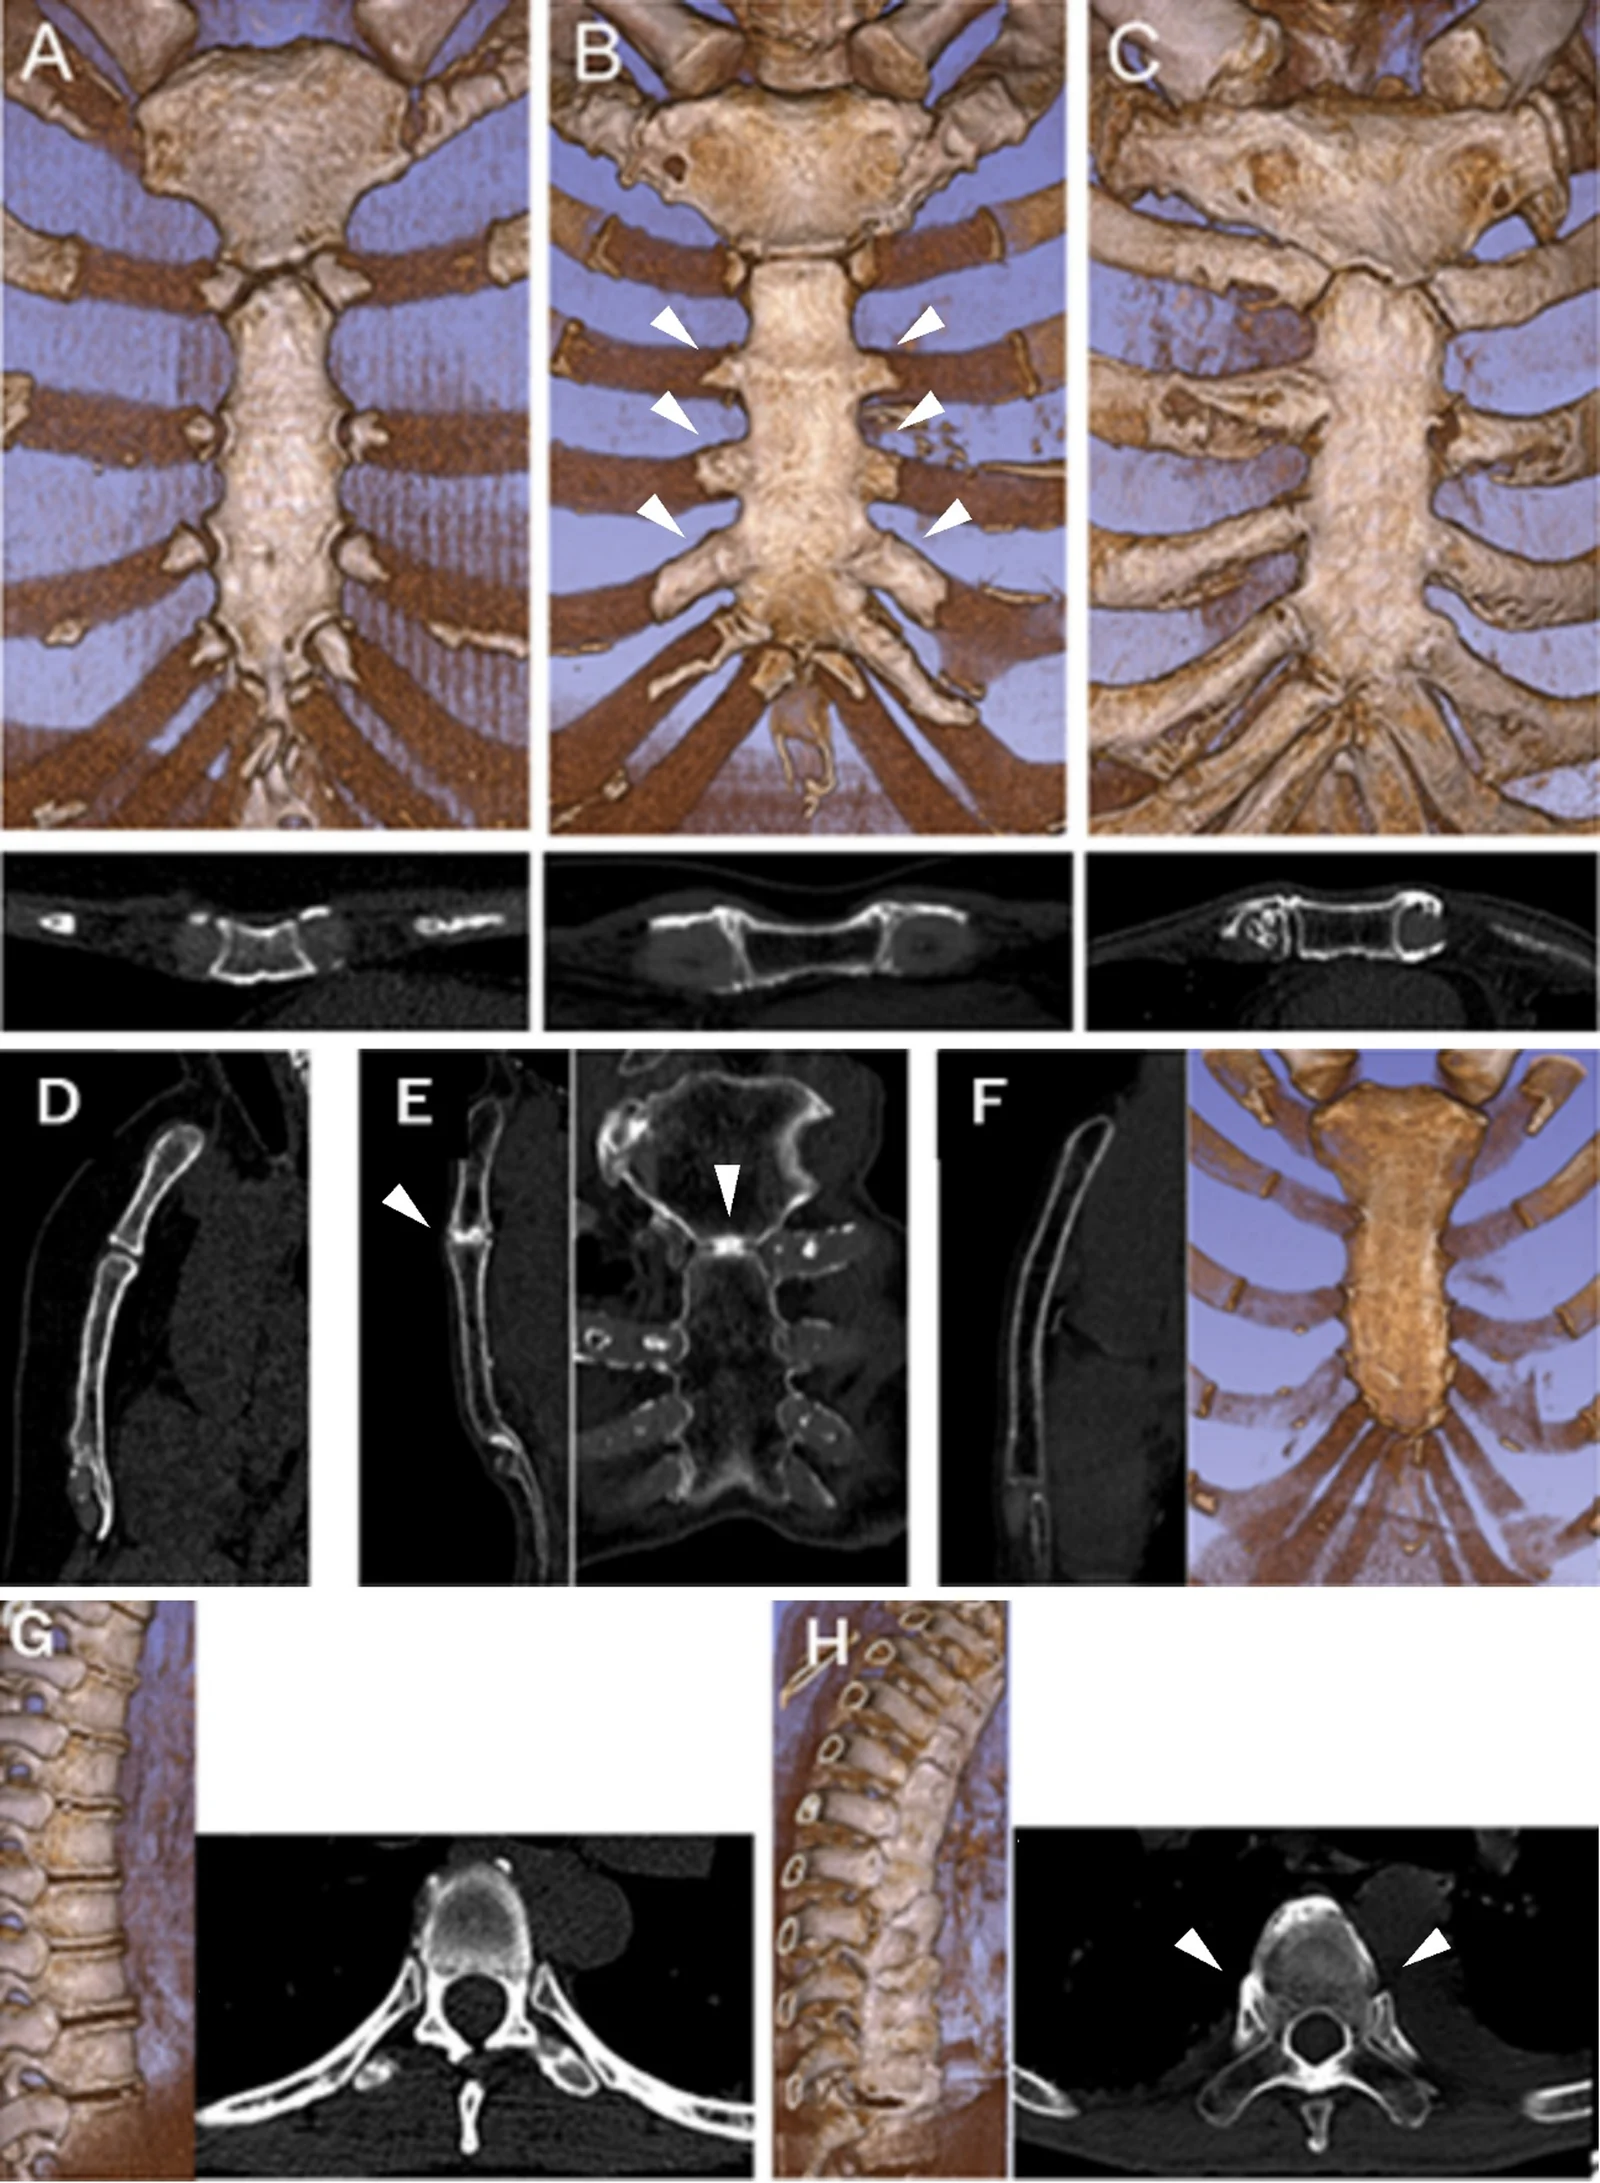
Representative cases of thoracic joint abnormalities in IPPFE and non‑PPFE ILDs. A Normal sternocostal joints (SCJs) in a case of fibrotic hypersensitivity pneumonitis (fHP; #FHP2). Mild ossification of the costal cartilage was observed; however, the SCJs remained intact. B Calcified SCJs of the third to fifth ribs in an IPPFE case (#IPPFE3) (white arrowhead), where calcified ligaments bridge the sternal body and costal cartilages. C Extensive calcification of the SCJs with ossified costal cartilages in an IPPFE case (#IPPFE4), resulting in a thoracic cage resembling an immobile armor. Frontal view by volume rendering (upper) and horizontal CT image at the fifth SCJ with the bone window setting (lower) are shown. D Normal manubriosternal joint (MSJ) in an fHP case (#FHP2). E Calcification of the MSJ in an IPPFE case (#IPPFE2) (white arrowhead). F Complete fusion of the MSJ with severe asymmetry of the 2nd–4th SCJs and cartilages in an IPPFE case (#IPPFE5). G Normal costovertebral joints (CVJs) in an fHP case (#FHP2). H Abnormal CVJs in an IPPFE case (#IPPFE4), showing marked vertebral deformity and adhesion of thoracic vertebral column (left) and the sixth CVJ on axial view (right). Thoracic spine deformity and joint fusion due to the loss of articular space and calcification are noted (white arrowhead)MSJMSJ abnormality was found in 4/17 (24%) patients with IPPFE and in 0/15 patients without PPFE. Figure 2D shows a normal MSJ in a case of fHP (#FHP2). Figure 2E shows a calcified MSJ in an IPPFE case (#IPPFE2). Complete, non-calcified fusion between the manubrium and the body was found in 4/17 (24%) patients with IPPFE and 3/15 (20%) patients without PPFE, a frequency similar to that reported in Ref. 17. Of these, an IPPFE case (#IPPFE5), shown in Fig. 2F, exhibited severe asymmetry of the SCJ positions and costal cartilage orientation, which may cause abnormal thoracic motion. Therefore, we judged this case as having an abnormal SCJ.CVJsCVJ abnormality was found in 2/17 (12%) patients with IPPFE and in 0/15 patients without PPFE. Figure 2G shows normal CVJs (#FHP2), and Fig. 2H shows abnormal CVJs (#IPPFE4), in which marked deformation of the thoracic vertebral column and a horizontal cross-sectional view of the fused sixth CVJ are observed.
